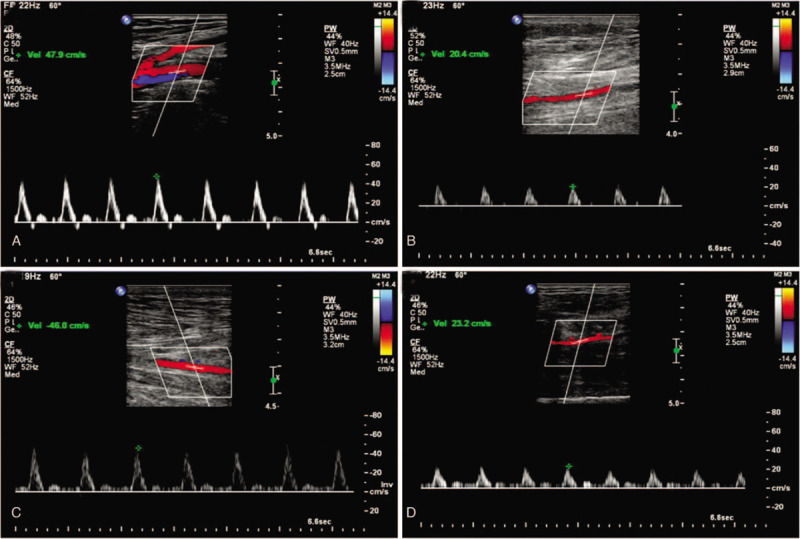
Six-month follow-up ultrasonographic images of popliteal artery (A), ATA (B), PTA (C) and peroneal artery (D). ATA = anterior tibial artery, PTA = posterior tibial artery.
